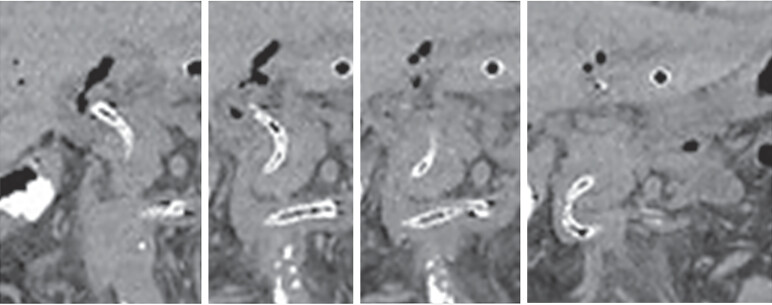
Computed tomography performed the following day demonstrated
